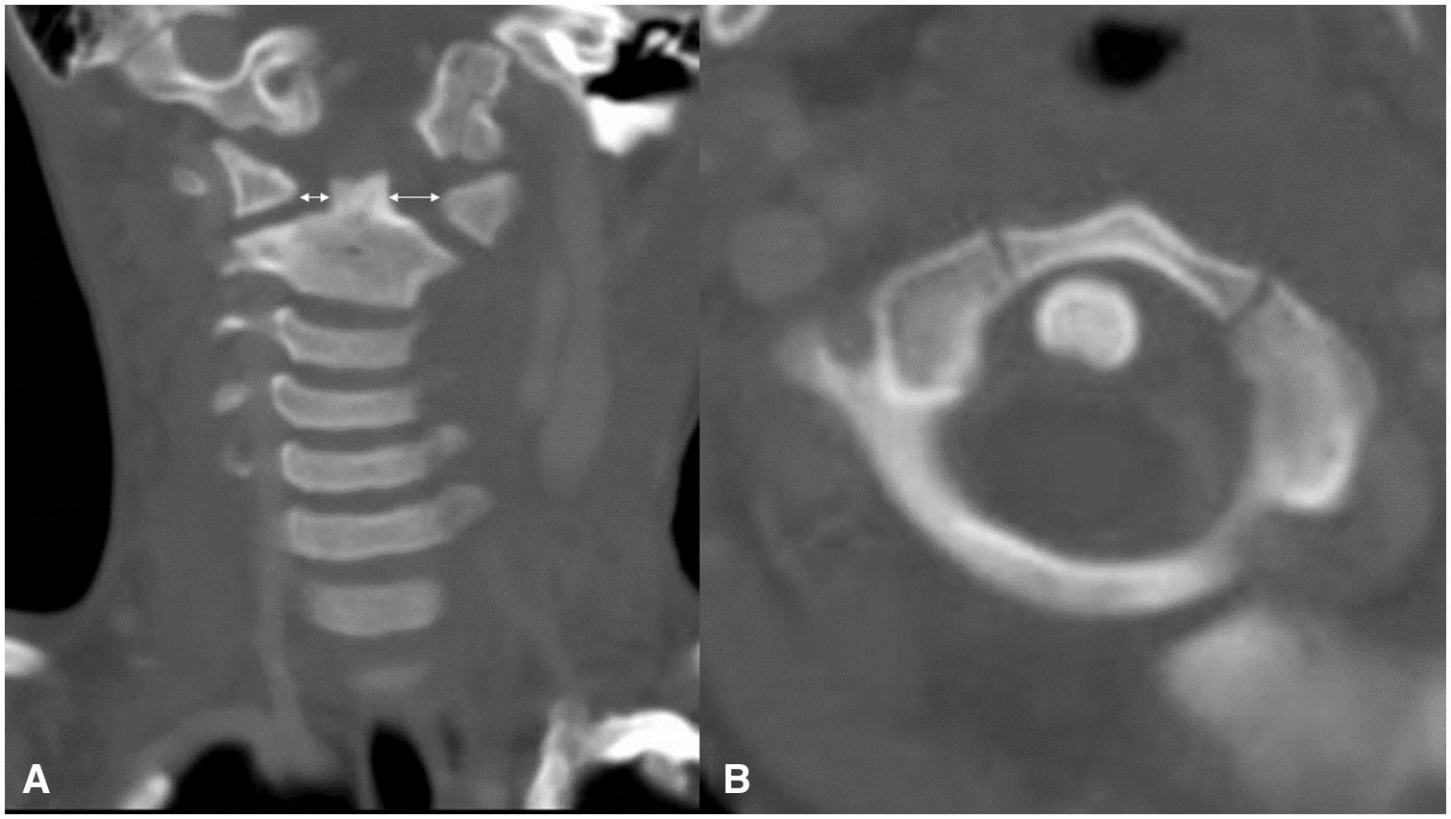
CT scan of the cervical spine. (A) Coronal CT image showing a gap on the left C1-C2 joint. (B) Axial CT image showing atlantoaxial subluxation of Fielding classification type I.
